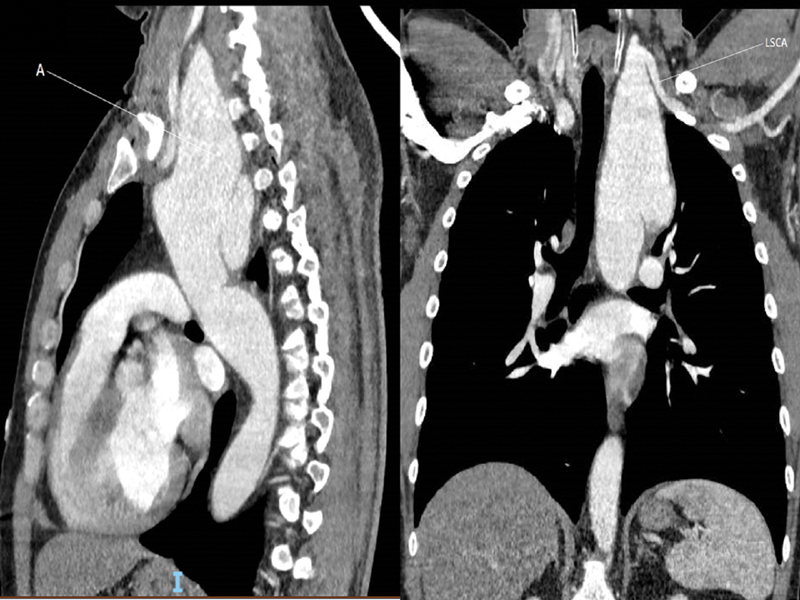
Computed tomography scan of patient 1. A,aneurysm ; LSCA,left subclavicular artery.
